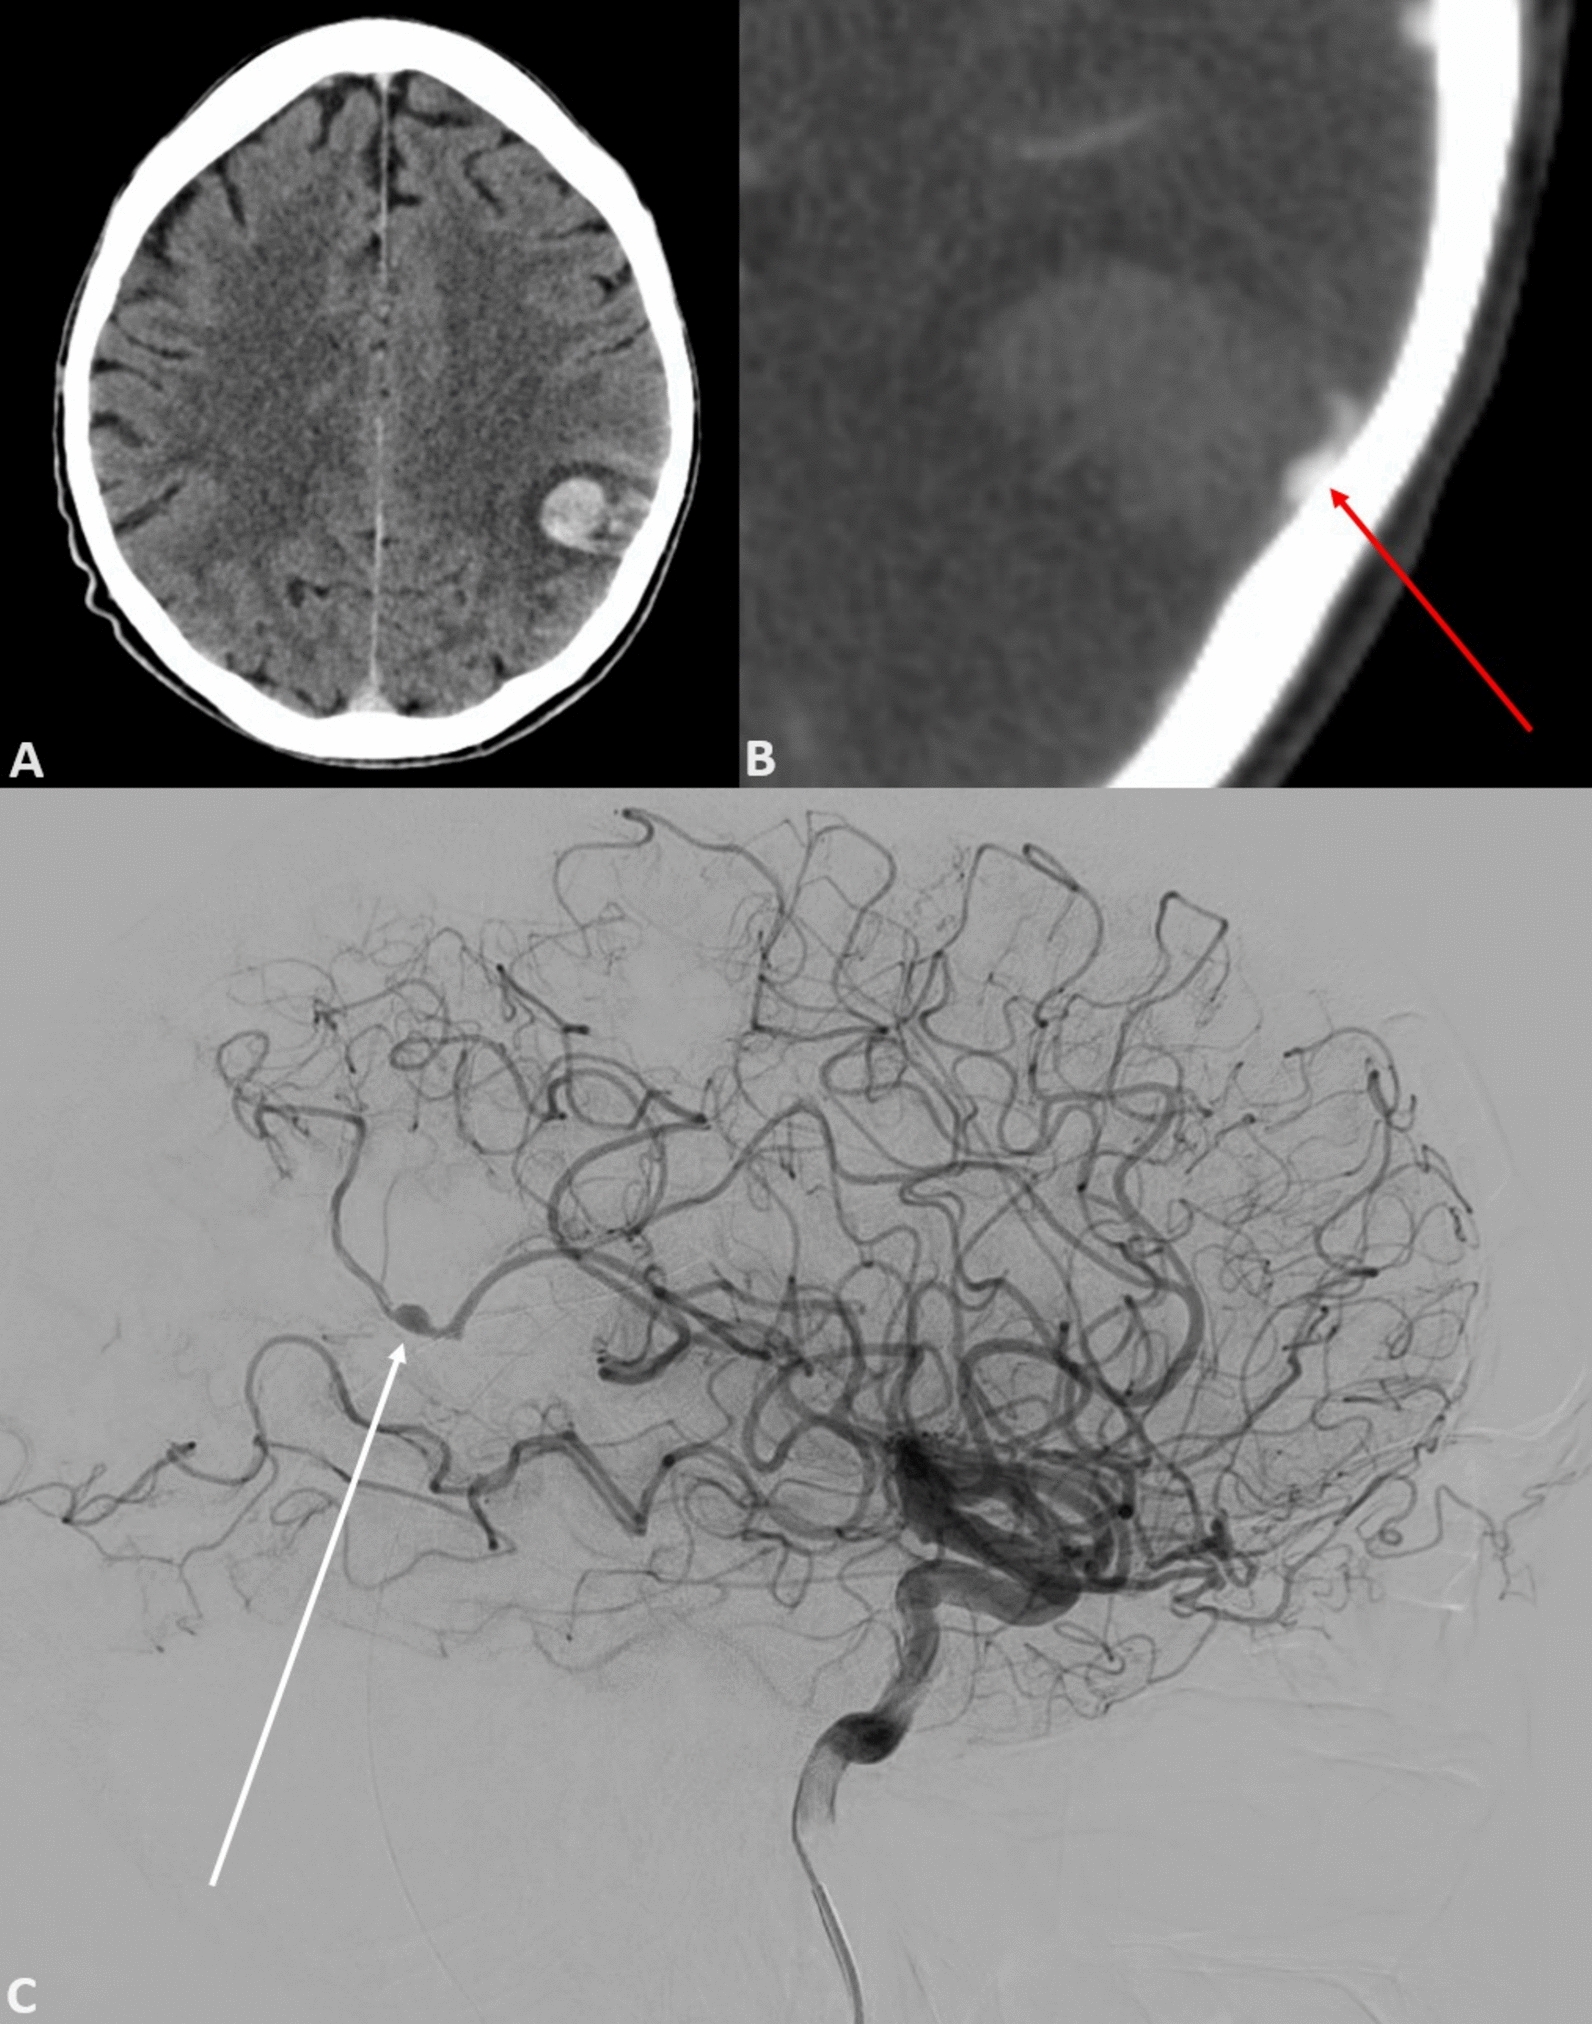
From top right moving clockwise; image A showing slice of a non-contrast contrast tomography brain scan showing a left parietal subarachnoid haemorrhage; image B showing computed tomography angiogram revealing a left middle cerebral artery branch vessel fusiform aneurysm; image C showing still from a digital subtraction angiogram revealing a pseudoaneurysm in relation to the superior parietal branch of the left middle cerebral artery
